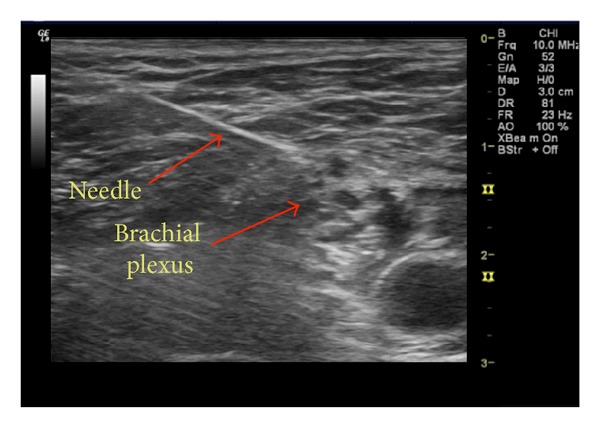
Ultrasound image of low interscalene block.
